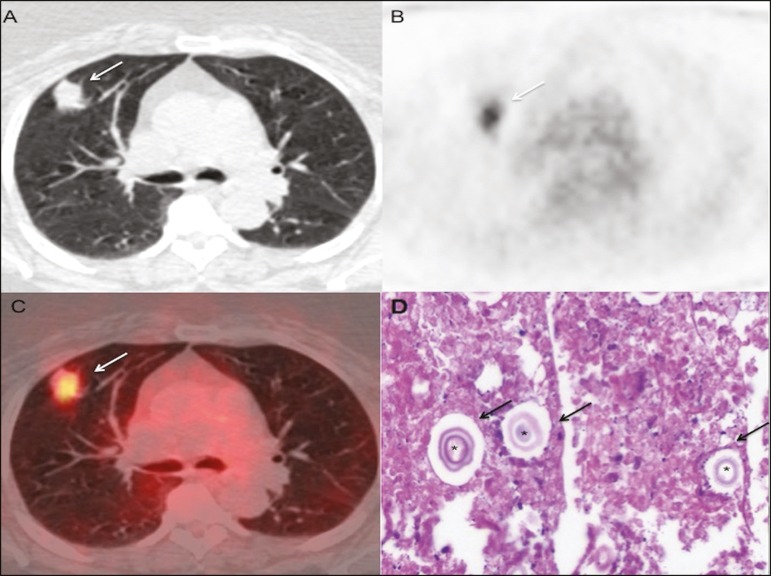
A-C: PET/CT scans showing aspiculated mass with soft tissue
